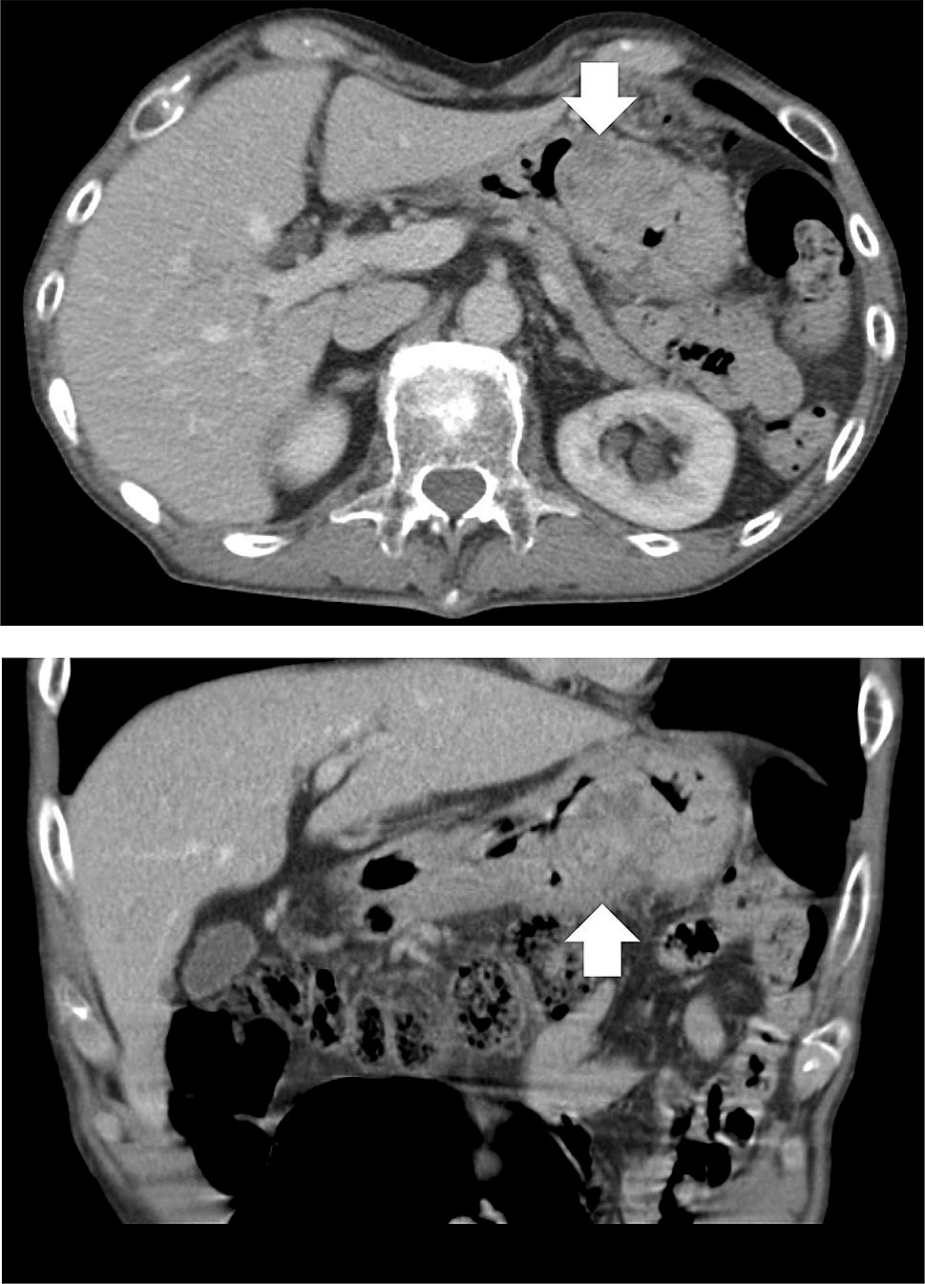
Computed tomography shows tumor with an unevenly contrasted surface of approximately 50 mm in diameter along the stomach wall (white arrows)
